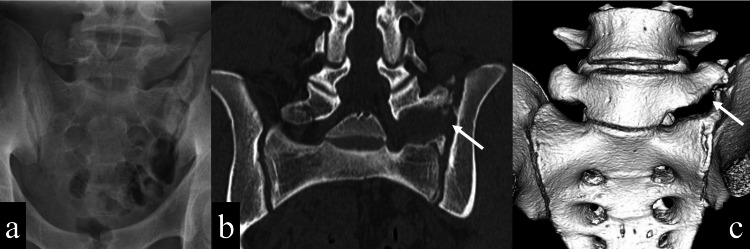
Immediate postoperative imaging findings a: Plain X-ray anteroposterior view showed dissection of the bony continuity of the transverse process and the sacral alar or iliac crest. b: CT coronal view image showed adequate bone resection (white arrow). c: CT 3D image showed dissection of the bony continuity between the transverse process and the sacral alar or iliac crest (white arrow).
